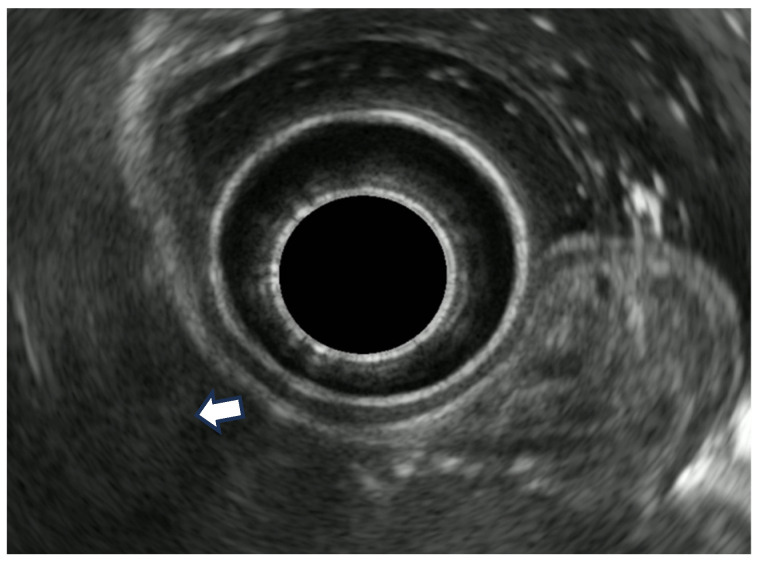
Ectopic pancreas in the III layer, with anechoic structures (arrow) without Doppler signal (pancreatic ducts).
